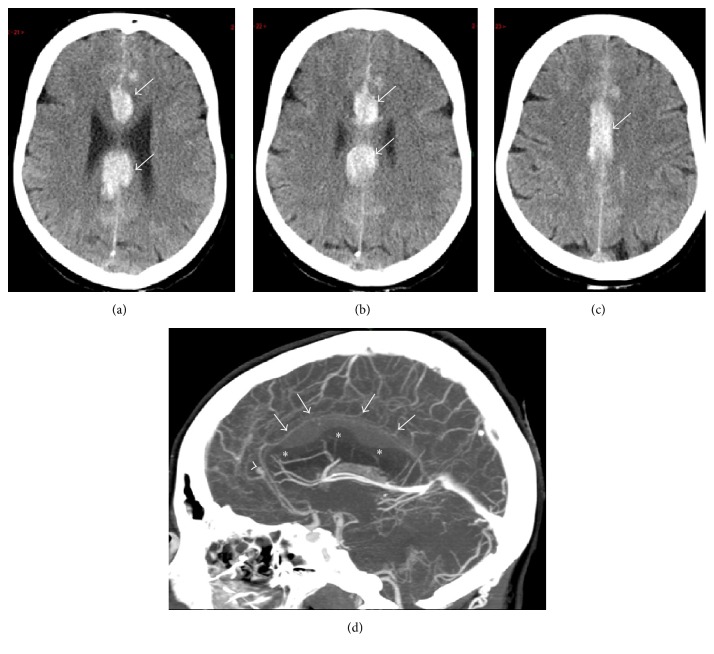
Admission brain CT scanner work-up of patient 1. (a–c) Serial unenhanced axial transverse views through the level of the corpus callosum showing massive hyperintense subpial hematoma at the cranial border of the corpus together (white arrows) with a mild SAH at the lateral borders of the frontal and parietal lobes, mainly on the left side. (d) Contrast-enhanced mid-sagittal reformatted Maximum Intensity Proton (MIP) view showing severe polylobulated impingement of the subpial hematoma (white arrows) on the corpus callosum (asterisks) and the causative microaneurysm on the left ACA (arrowhead). Note artifactual lowering of the apparent density of the hematoma because of changes in image scaling (window/level) after contrast agent injection.
